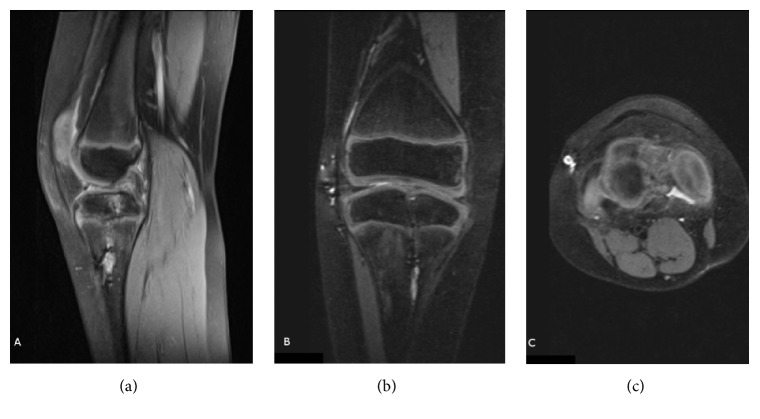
Postoperative MRI (sagittal (a), coronal (b), and transverse (c) views) of the left knee of the patient from Figures 1–3. Graft integrity was inconclusive.
